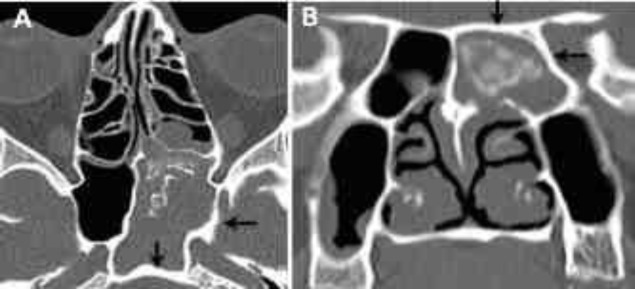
Axial computed tomography scan (A) showing complete opacification of the left sphenoid sinus with associated sclerosis of its bony walls (black arrows), and multiple foci of microcalcifications. On coronal computed tomography scan (B), there is a hyperattenuating rounded mass in the left sphenoid sinus consistent with a  fungus ball
